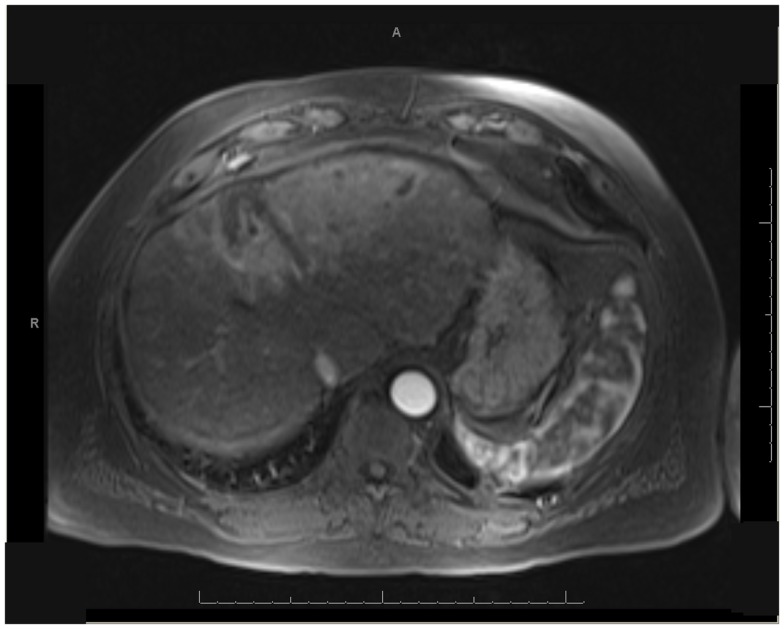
T1 MRI with early phase contrast. Seven weeks post-RE.
